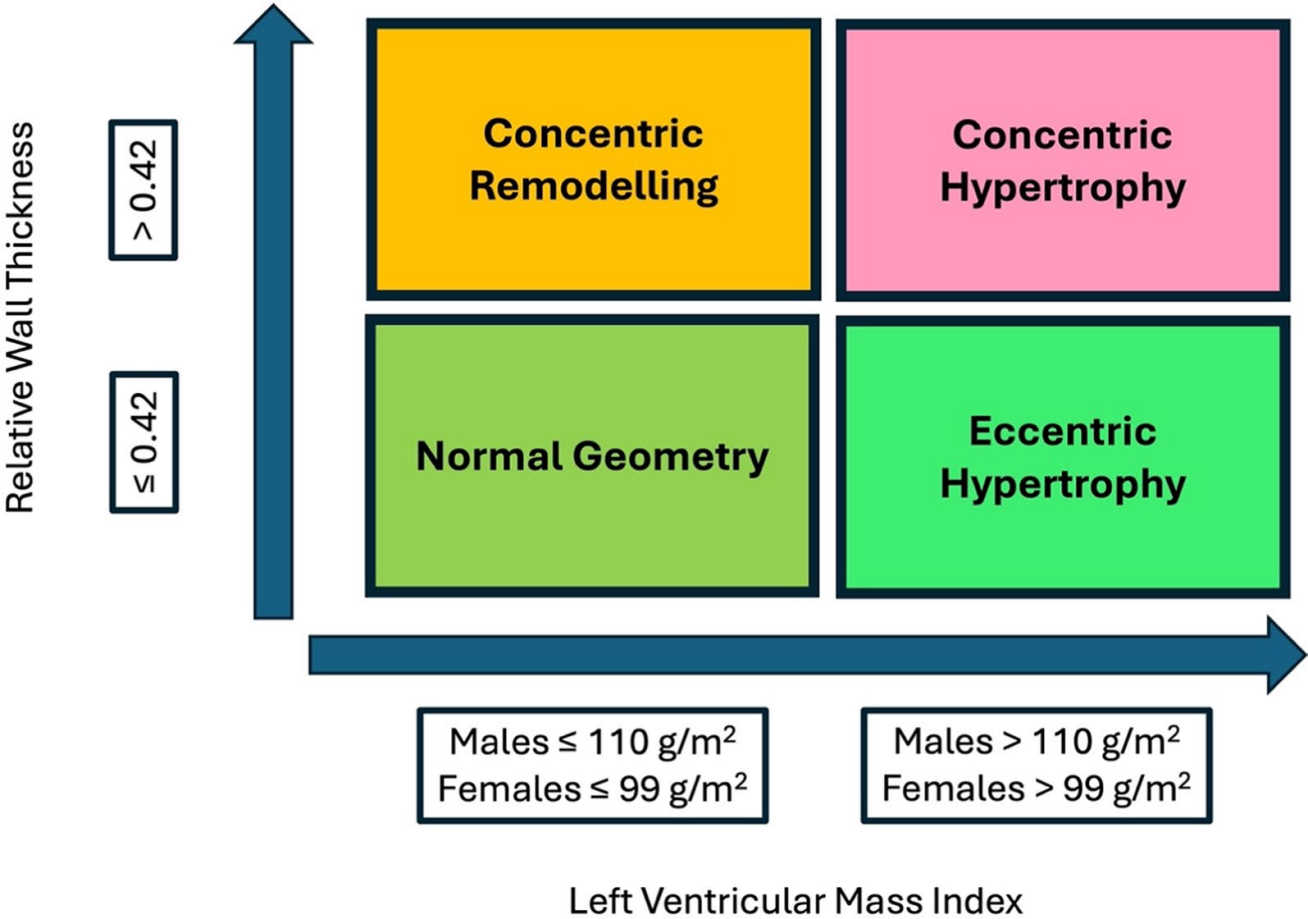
Defining LV Geometry using Standard 2-dimensional Echocardiography
